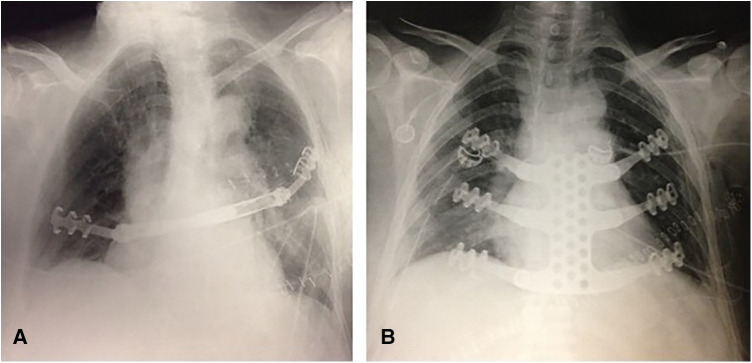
Postoperative chest x-rays after (A) Thorib® bar interposition and (B) Trionyx® sternal reconstruction.
